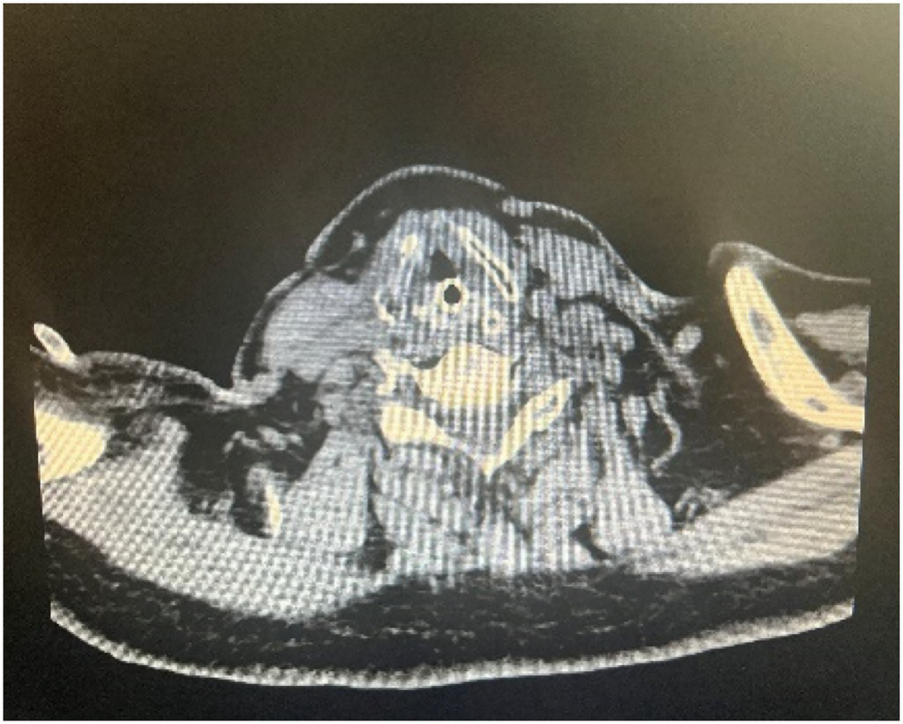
Postoperative cervical CT findings. No obvious stenosis or obstruction was observed in the glottic region, and the tracheal morphology was normal.
